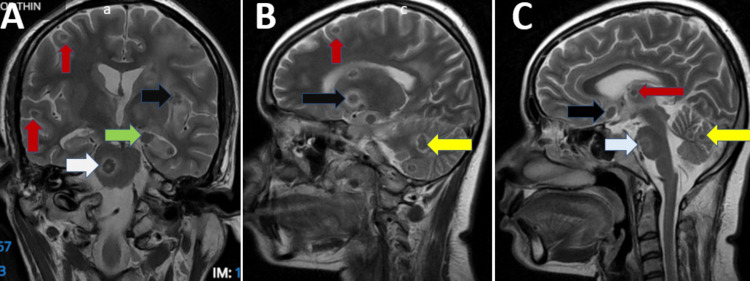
T2-weighted MRI of the brain showing coronal (A) and sagittal (B, C) projection Hypointense ring-enhancing lesions marked with arrows in (A) gray-white matter junction (red), right pons (white), conglomerate lesions near the left insular cortex (black), and left hippocampus (green); (B) cerebellum (yellow), gray-white matter junction (red), and basal ganglia (black); and (C) thalamus (red), medial temporal lobe near the optic chiasm (black), pons (white), and cerebellum (yellow).
